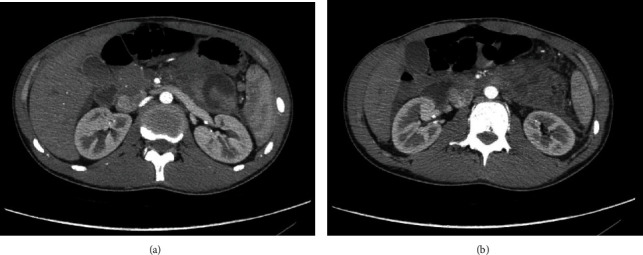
CT scan on the 2nd day posttrauma. (a) Peripancreatic blood collection (3.5 cm). (b) Increase of fluid collection peripancreatic, perisplenic, and along the left lateral conal fascia.
